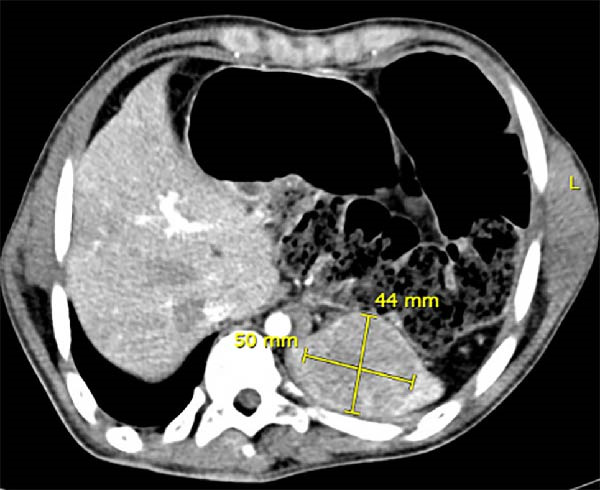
Computed tomography axial section of upper abdomen showing splenic mass measuring > 4.5 cm.
